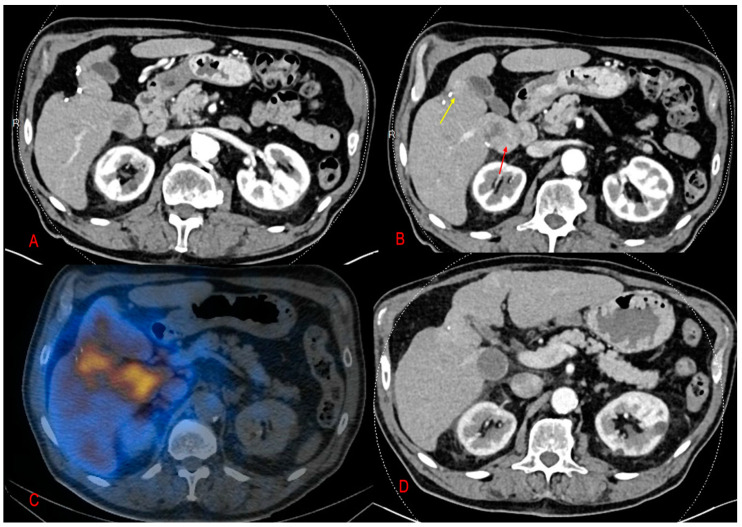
A 63-year-old man with recurrent HCC following tumor resection in segment V. (A,B) CT scan in arterial phase showing two hyper-vascular lesions, one in the segment I (red arrow) and the other beside the surgical clips (yellow arrow). (C) Fused images of PET-CT obtained 24 h following the treatment session of SIRT showed intense concentration of microsphere in the corresponding hepatic tumor (the orange content). (D) CT scan after five months of SIRT demonstrated a complete tumor regression.
